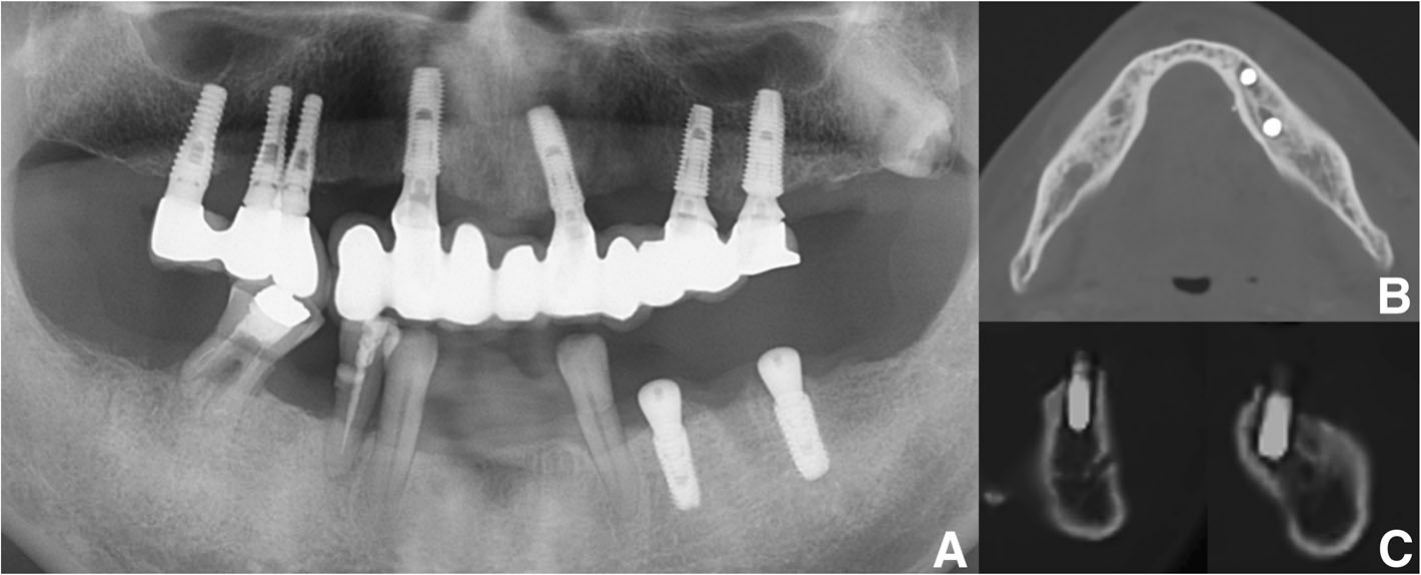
a Panoramic view: dental implant installation state on mandible Lt. Posterior area (bleeding area). b Axial CT view and c sagittal CT view on implant site showed adequate placement of dental implant without lingual cortex perforation and damage of adjacent anatomic structure. The dental implant installation in the left posterior mandibular area
